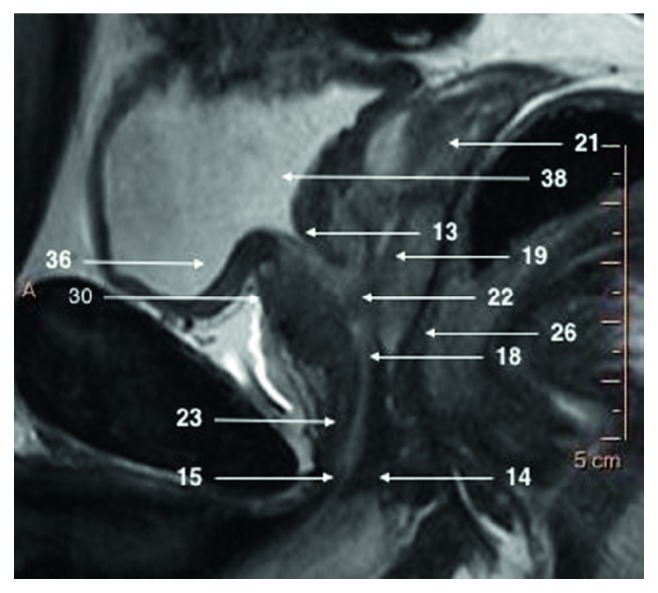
T2-weighted sagittal image focused on a prostate gland.
